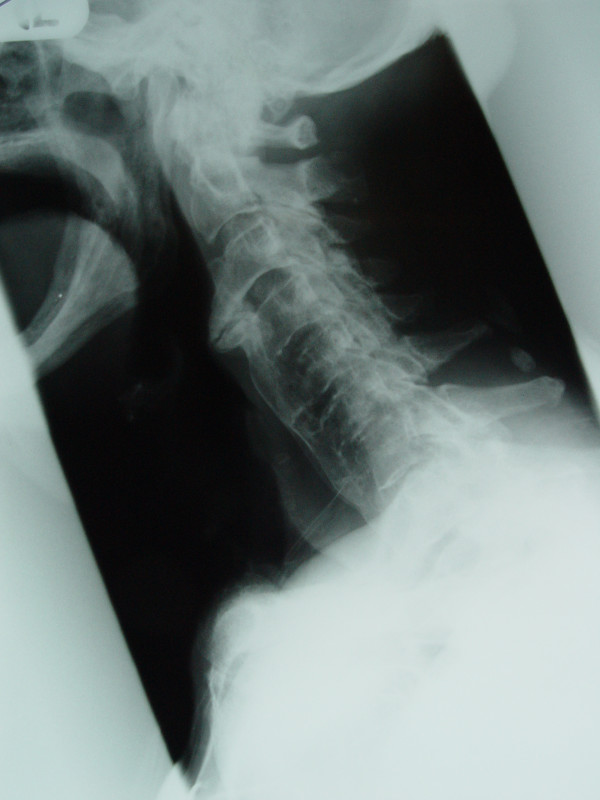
Lateral radiograph of the cervical spine showing anterior osteophyte formation most marked at the C3/C4 vertebrae and calcification of the anterior longitudinal ligaments.
